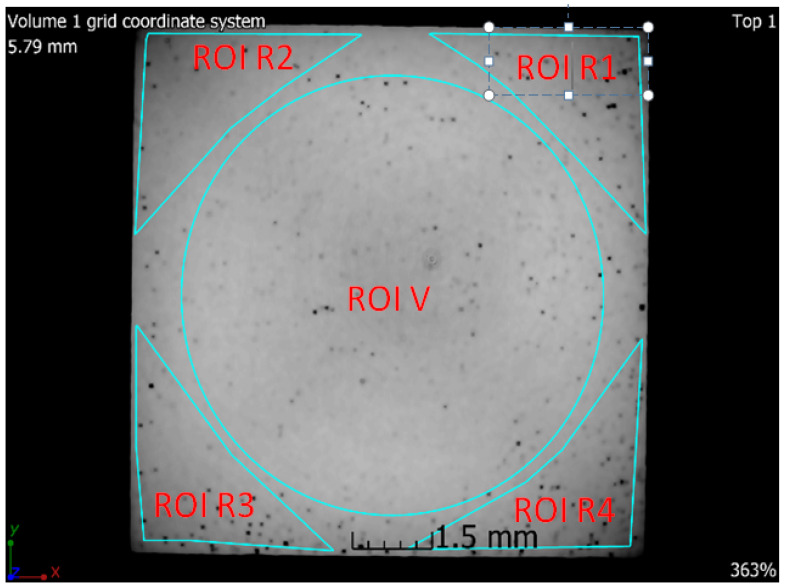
Tomographic CT sections of sample 5 with marked ROIs (regions of interest).
